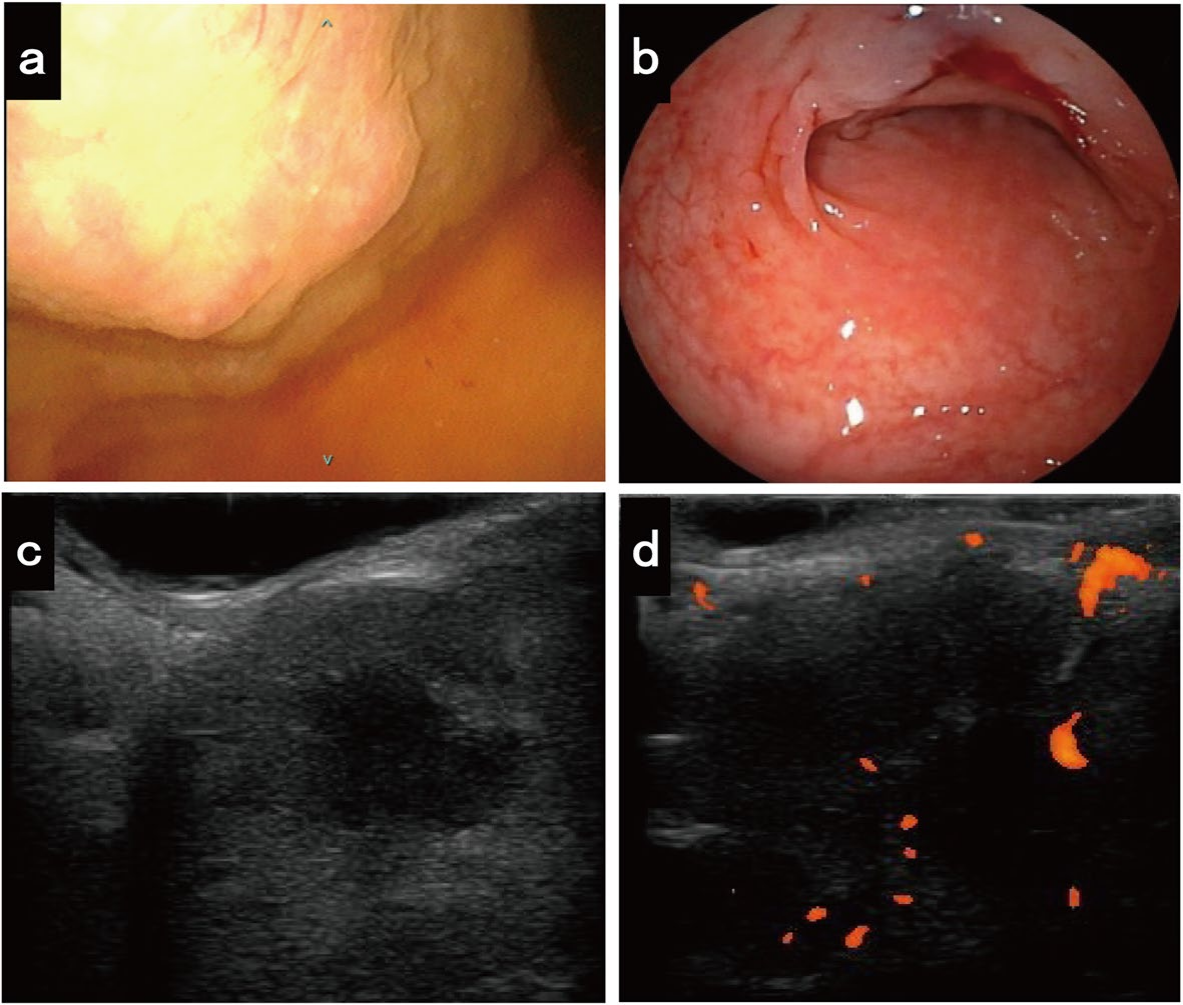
Findings of cystoscopy, colon fibroscopy, and endoscopic ultrasonography. a Cystoscopy showing compression of the trigone of the bladder by external masses, but no visible tumor in the lumen. b Colon fibroscopy showing compression of the anterior rectal wall and no tumor in the tract. c Endoscopic ultrasonography revealing clearly defined hypoechoic mass localized in the anterior wall of the rectum. d Doppler-echo showing that the mass is well vascularized at the periphery
